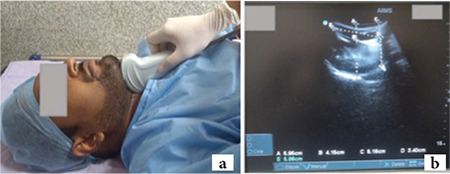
a) First step Airway-USG-patient head in neutral position with curvilinear transducer placed in suprahyoid region at the midline in sagittal plane, adjusted to bring the hyoid bone, muscles of floor of the mouth, the entire tongue and mentum in one frame. b) Ultrasonographic image at first step. A=Skin to tongue distance-maximum, B=Tongue thickness-maximum, C=Skin to tongue distance at base, D=Tongue thickness at base of tongue, E=Hyomental distance in neutral (Note hyperechoic air-mucosa interface at dorsum of tongue). USG, ultrasonography.
